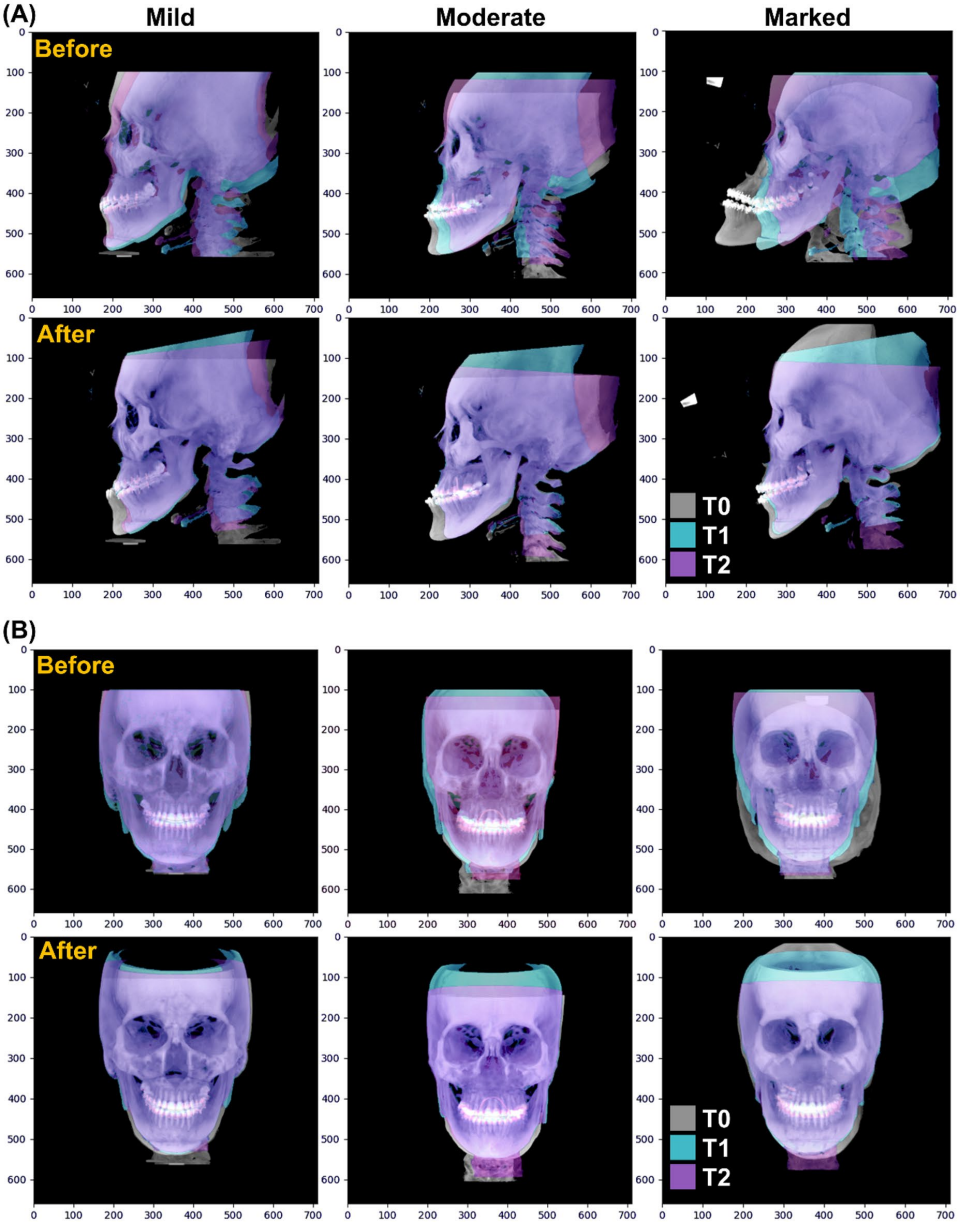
Qualitative visualization of the serial cone beam computed tomography volume. The columns represent three clusters of clinical asymmetries, i.e., mild, moderate, and marked. The (A) profile and (B) frontal images from three-time points (T0, T1, and T2) are presented as an overlay. The overlay images show an improvement in goodness after the proposed principal coordinate-based alignment is performed.
